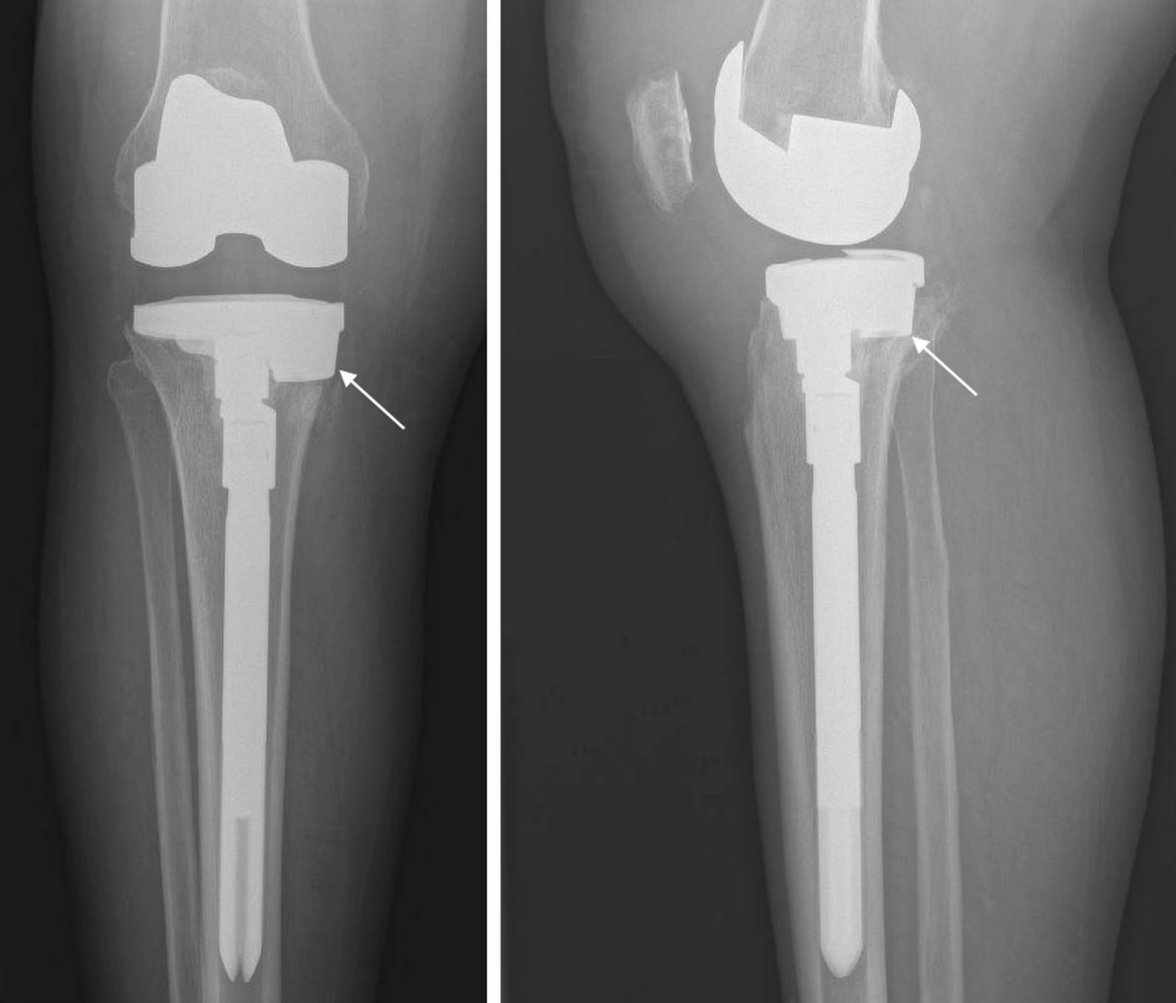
AP (left) and lateral (right) radiographic imaging demonstrating a medial tibial augment (arrow) and hybrid fixation with metaphyseal cementing, an offset connector, and a cementless tibial stem
